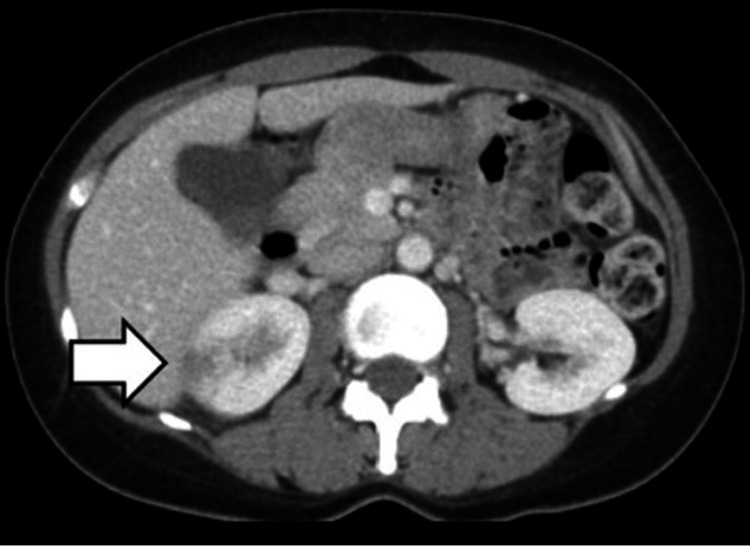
A contrast-enhanced computed tomography image showing a wedge-shaped, partially contrast-impaired area in the right kidney
